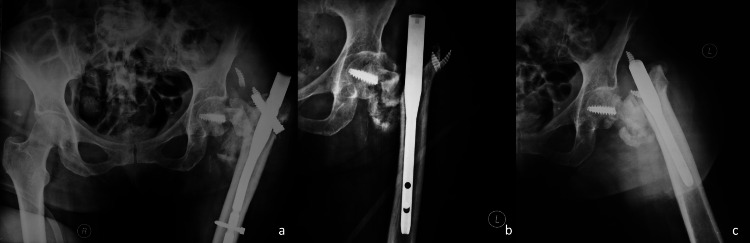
Anteroposterior pelvis (a) and lateral left hip (b,c) x-rays after implant failure.
